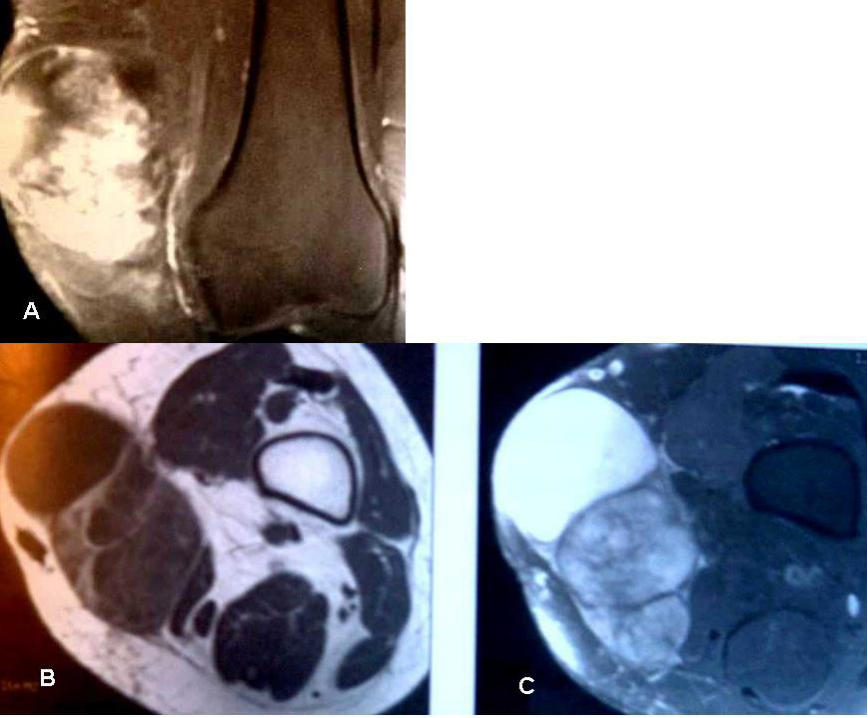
MRI: 3A, 3B: MRI-Scan of myxoid liposarcoma of the thigh, coronal (3A) and axial (3B) views.
